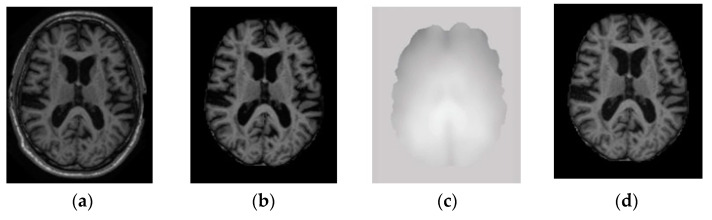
Pre-processing of MRI. (a) T1-W original MRI, (b) Brain tissue image after removal of the nonbrain structure, (c) The bias field, (d) Brain tissue image after bias field correction.
